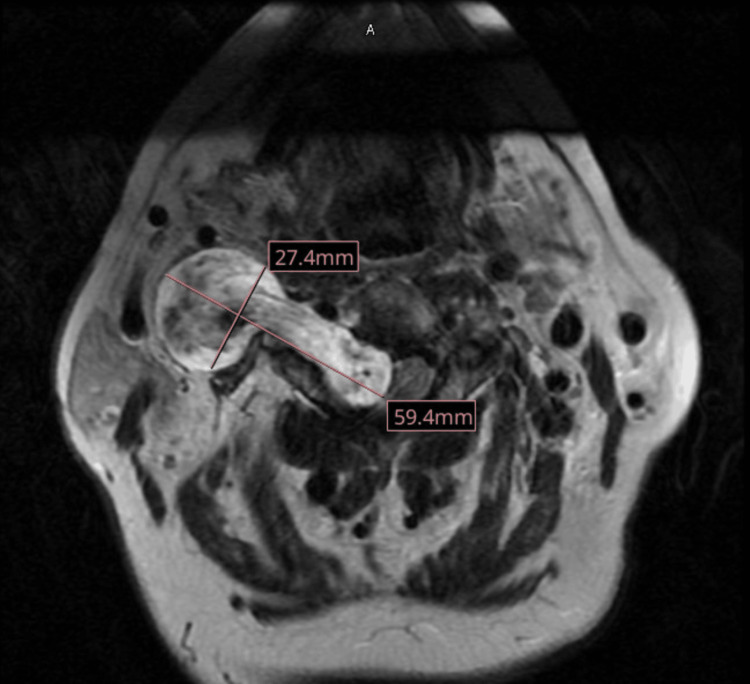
T2 weighted MRI image of schwannoma showing spinal canal stenosis and vertebral artery compression.
